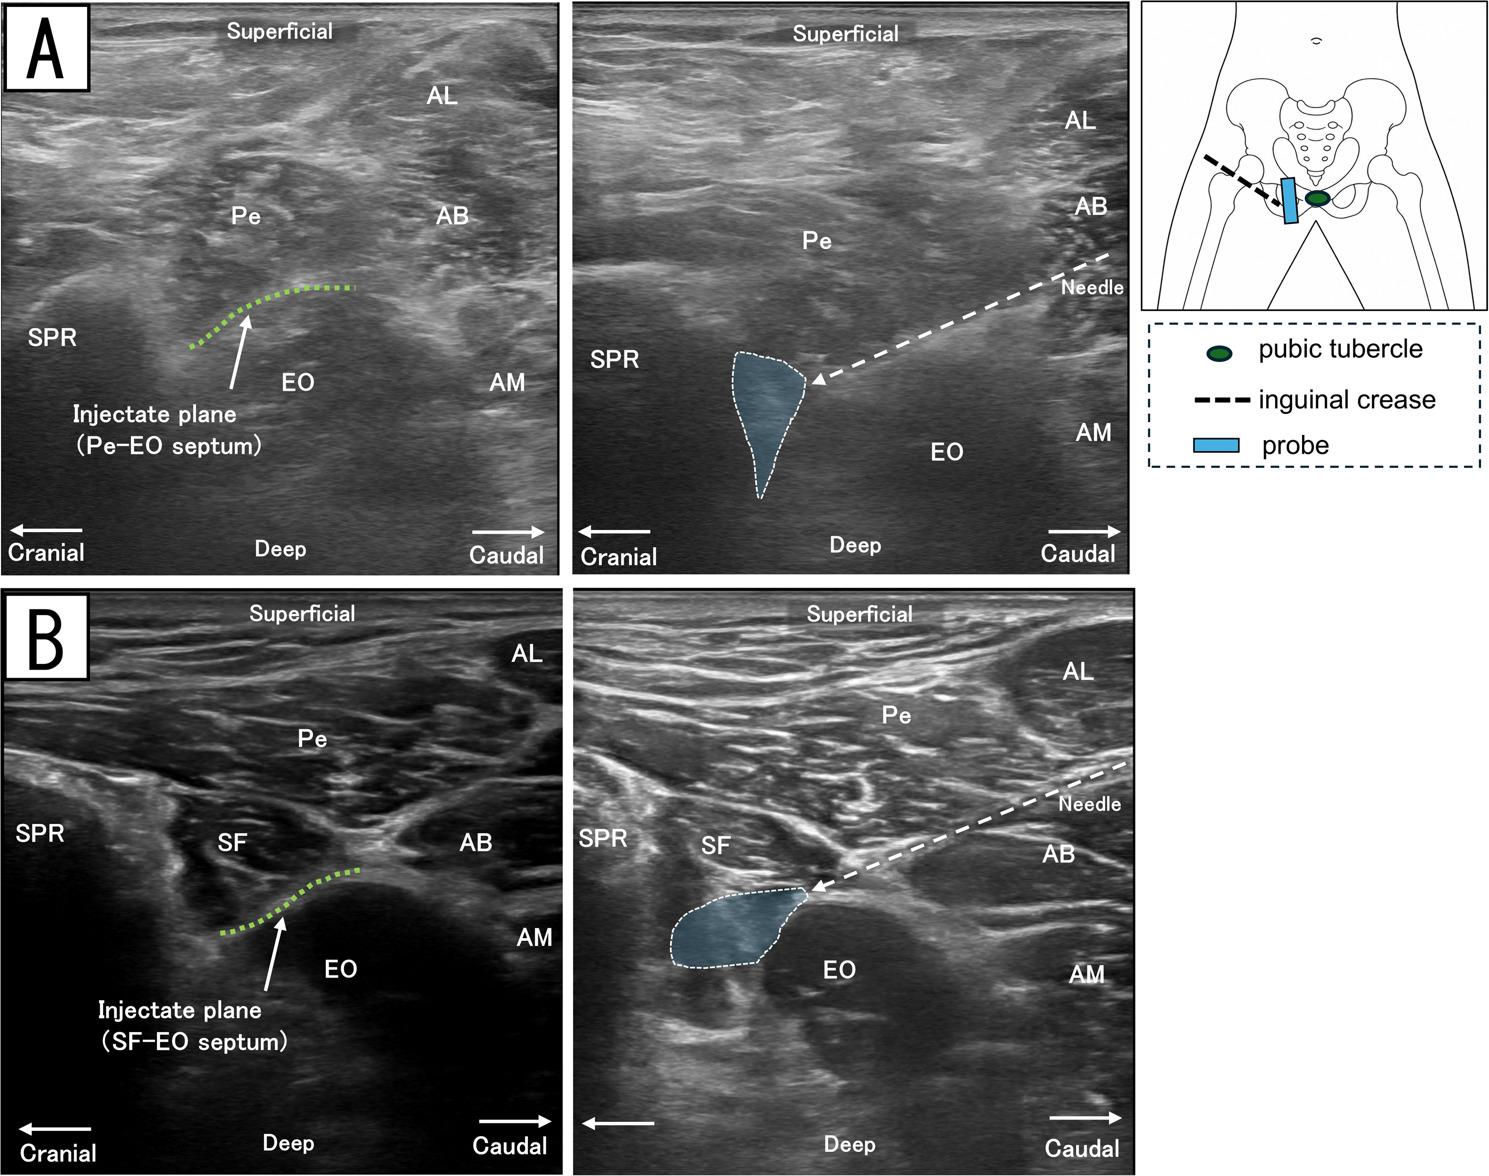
Ultrasound-guided interadductor approach for obturator nerve block. A No development of the superior fascicle of the external obturator muscle is observed. The needle is advanced in-plane toward the intermuscular septum between the pectineus muscle and the external obturator muscle. A mixture of local anesthetic and contrast medium is injected. AB, adductor brevis muscle; AL, adductor longus muscle; AM, adductor magnus muscle;Pe, pectineus muscle; EO, external obturator muscle; SPR, superior pubic ramus. B Development of the superior fascicle of the external obturator muscle is observed. The needle is advanced in-plane toward the intermuscular septum between the superior fascicle and the main body of the external obturator muscle. A mixture of local anesthetic and contrast medium is injected. AB, adductor brevis muscle; AL, adductor longus muscle; AM, adductor magnus muscle; Pe, pectineus muscle; SF, superior fasciculus of the external obturator muscle; EO, external obturator muscle; SPR, superior pubic ramus
